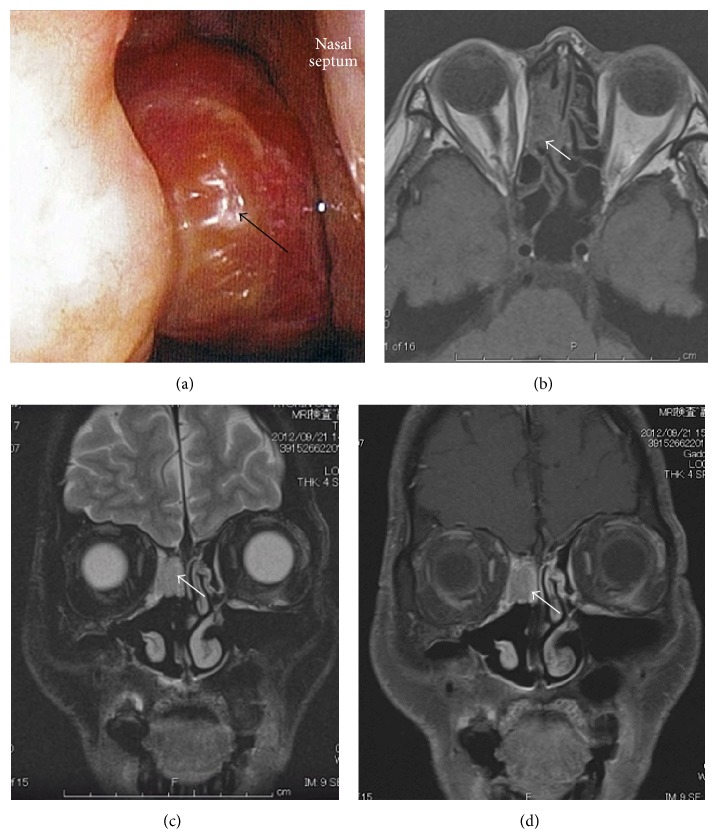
Preoperative local finding and MRI in case 2. (a) Tumor in the right nasal cavity showing a low-intensity area (black arrow). (b) T1-weighted axial image showing a slightly high-intensity area (white arrow). (c) T2-weighted coronal image (white arrow). (d) T1-weighted coronal image (gadolinium+) shows some enhancement within the lesion (white arrow).
